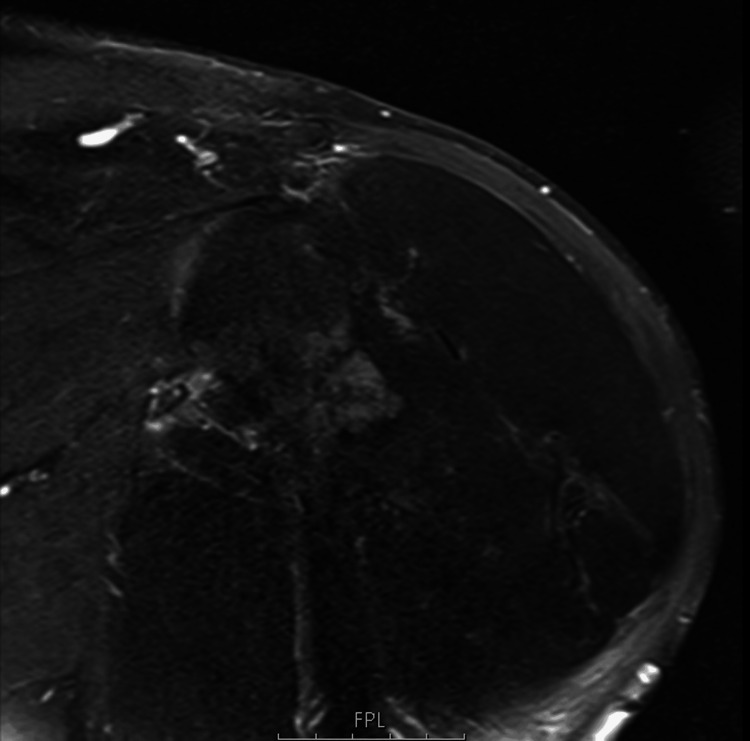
Coronal T2-weighted fat-saturated MRI. The lesion demonstrates low signal on T2 fat saturation, supporting the hypothesis of adipose content.
